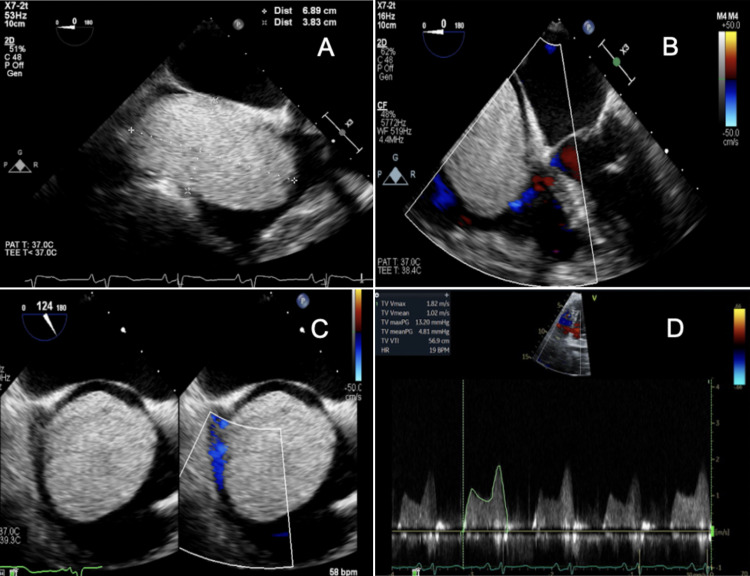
Echogenic Mass in Right Atrium Transesophageal echocardiogram (TEE) images showing the solid echogenic mass in the right atrium in different views (A-C) along with gradients across the tricuspid valve (D).
